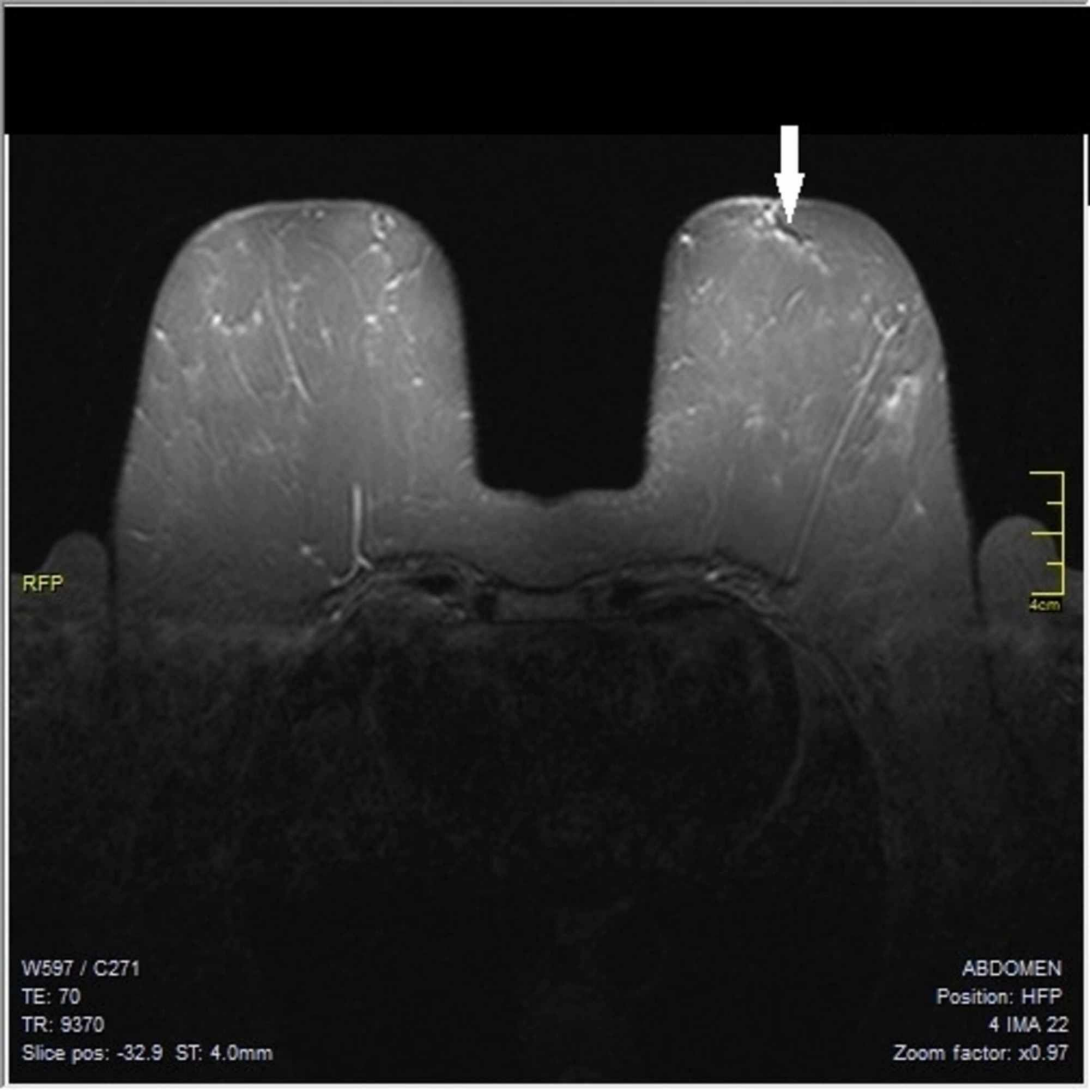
Magnetic resonance mammography (MRM) demonstrating a linear lesion, mammography and ultrasound (US) invisible
